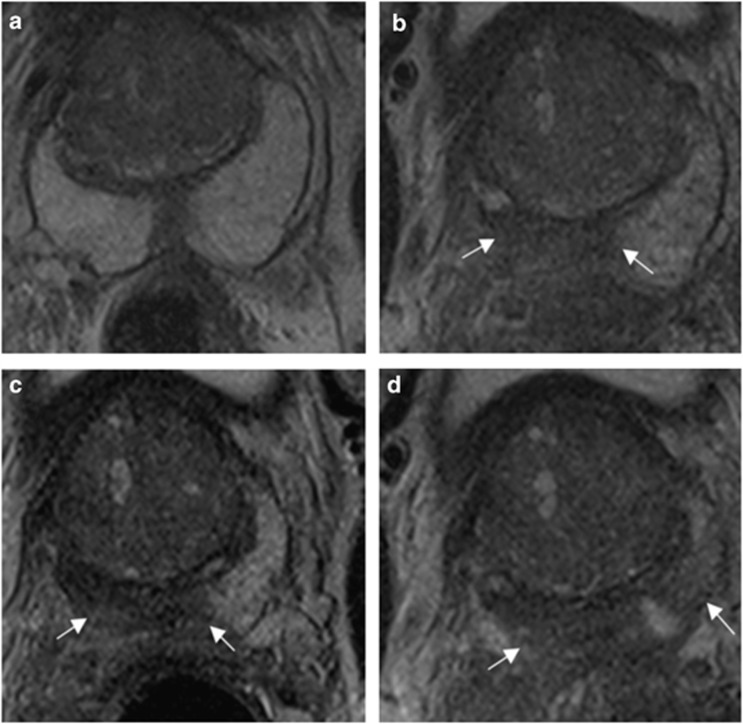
Representative axial T2-weighted images at the mid-gland demonstrate (a) normal peripheral zone T2 signal intensity pre-biopsy; and reduction (white arrows) in the peripheral zone T2 signal intensity at (b) 1 month, (c) 2 months and (d) 6 months post biopsy.
